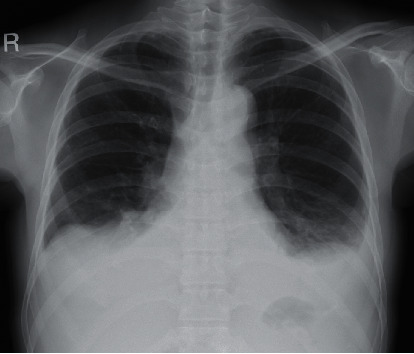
Chest X-ray on admission showing bilateral dullness of the costophrenic angles.
